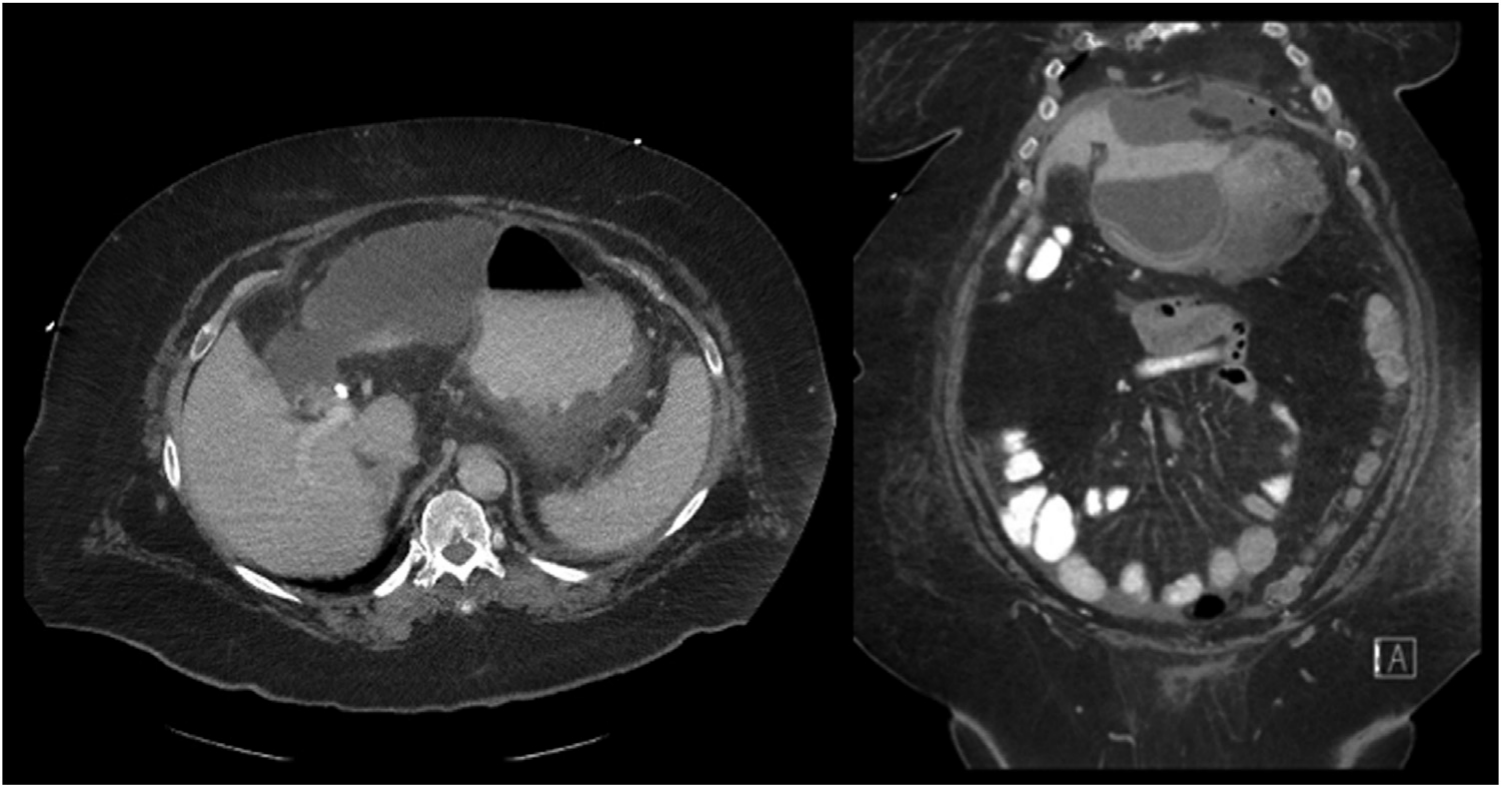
CT scan of abdomen showing the biloma.
